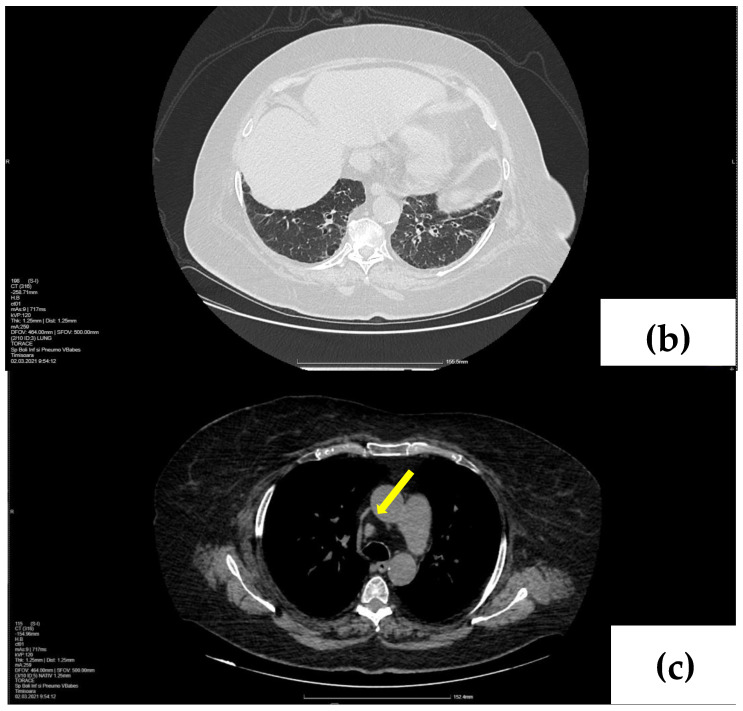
CT scan after treatment. Axial lung windows at the level of the middle lobe (a) and lower lobes (b) show a decrease in the RML mass and some scars in the lower lobes. (c) Right paratracheal (4R) lymphadenopathy involution (arrow).
